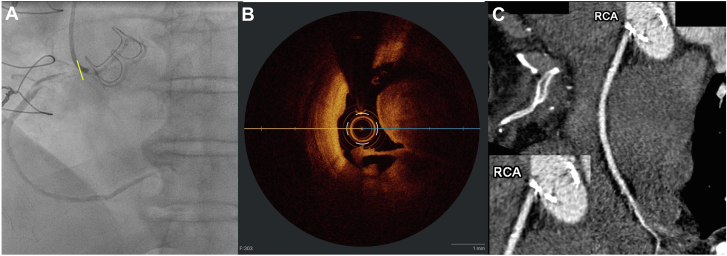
Images from elective aortic valve replacement postoperative day 7. (A) Angiogram of the subselected RCA showing an ostial filling defect, concerning for thrombus. The ostium appears to be behind the frame of the bioprosthetic aortic valve. The RCA was unable to be selected despite multiple attempts with different catheters. The yellow line indicates the approximate location of the OCT image. (B) OCT of the proximal RCA after balloon angioplasty, demonstrating a long length of thrombus. (C) Computed tomography coronary angiogram reconstruction of the RCA. The RCA appears to arise at or below the level of the valve sewing ring with at least part of the vessel covered, and contrast opacification of the coronary appearing to arise from the left ventricular outflow tract. Low-attenuation material at the ostium of the coronary may be related to thrombus or surgical material. Inset: magnification of the RCA ostium. OCT, optical coherence tomography; RCA, right coronary artery.
